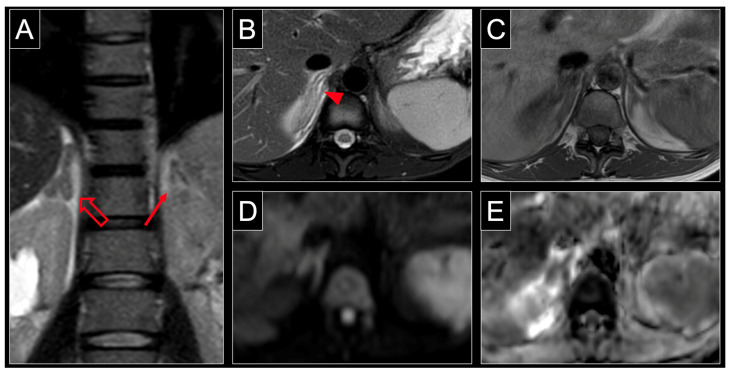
MRI of a 31-year-old patient at 34 weeks of pregnancy with non-hemorrhagic infarction of the right adrenal gland. Note the characteristic enlargement of the right adrenal gland (hollow arrow) compared to the normal contralateral adrenal gland (solid arrow) in the coronal plane of a T2 weighted sequence (A). Axial T2 weighting with fat saturation shows central and surrounding hyperintensity (arrowheads) reflecting organ edema with inflammatory response of retroperitoneal fat (B). The lack of intraparenchymal hyperintensity (arrowhead) in T1 weighting (C) indicates the absence of acute hemorrhage. Similar to other body regions, organ infarction is associated with restricted diffusion, which manifests as hyperintensity at high b-values, such as 800 s/mm^2^ (D) and low values in the ADC map (E) of DWI. ADC = apparent diffusion coefficient, DWI = diffusion weighted imaging.
